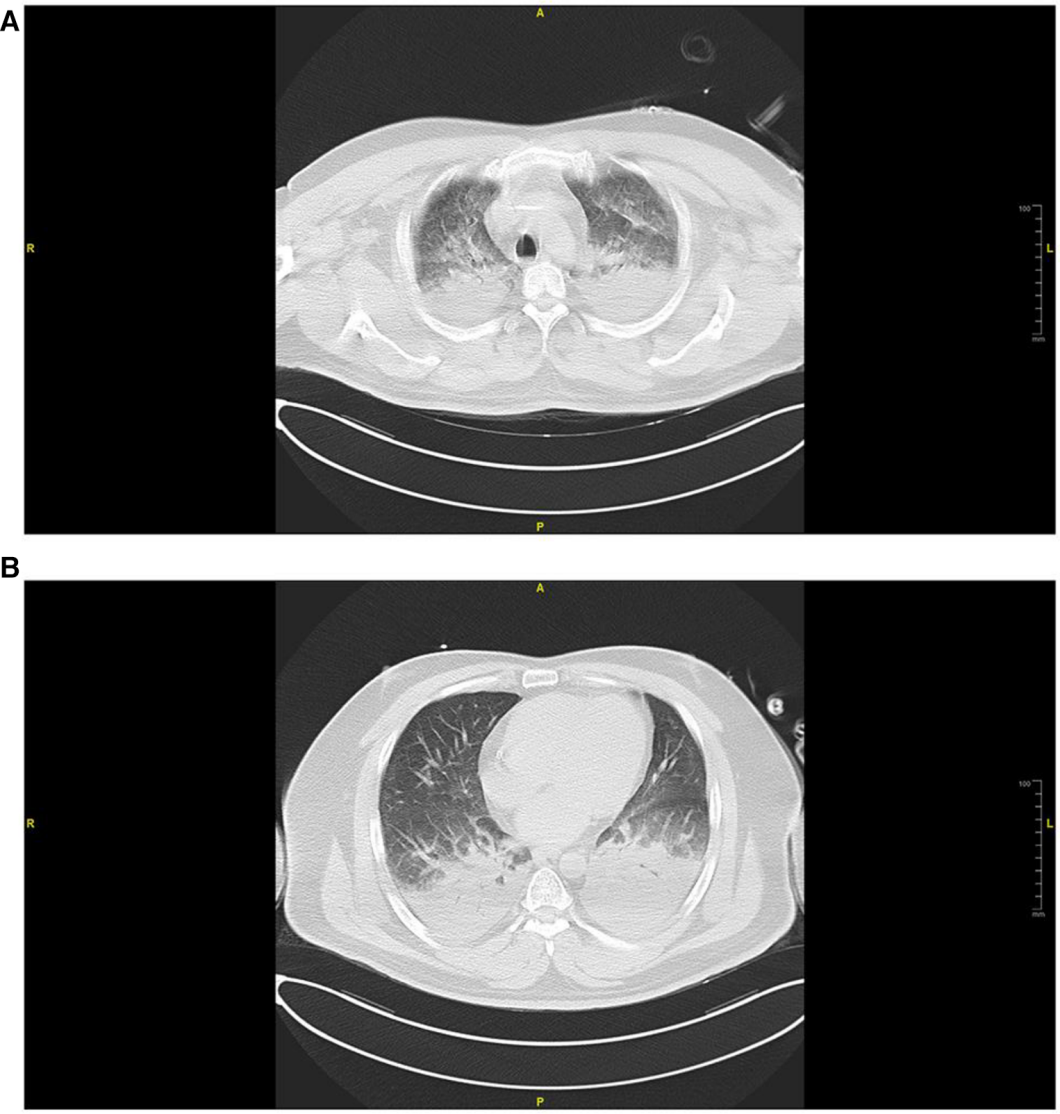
Chest CT scan conducted in the emergency room during admission. (A) Cross-sectional view of the aortic arch, (B) cross-sectional view of the mitral valve. Widespread pulmonary edema was noted bilaterally in both lungs.
